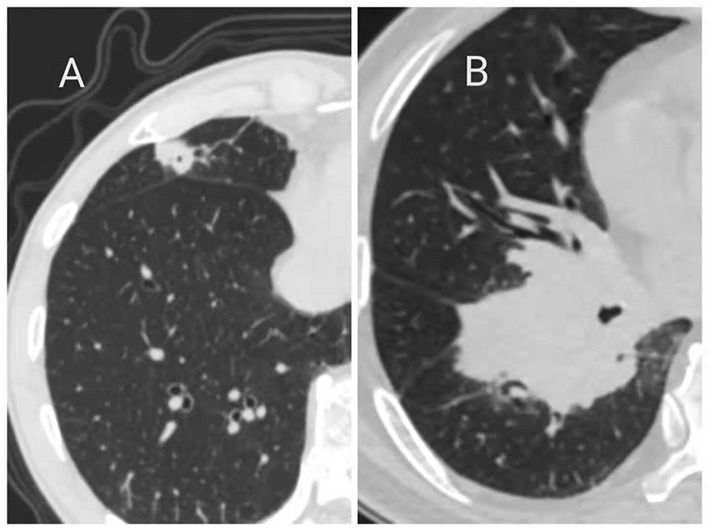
Primary pulmonary MALT lymphoma showed peripheral (A) and central (B) types on CT Imaging.
